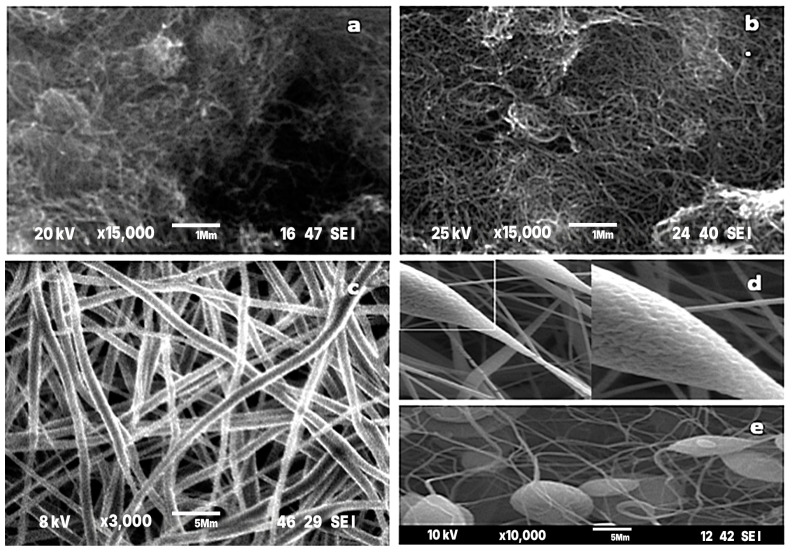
(a) CNTs before ultrasonic bath, (b) CNTs after ultrasonic bath, (c) Membrane of electrospun pure polycaprolactone fibers, (d,e) PCL nanofibers with the nanotubes embedded.
